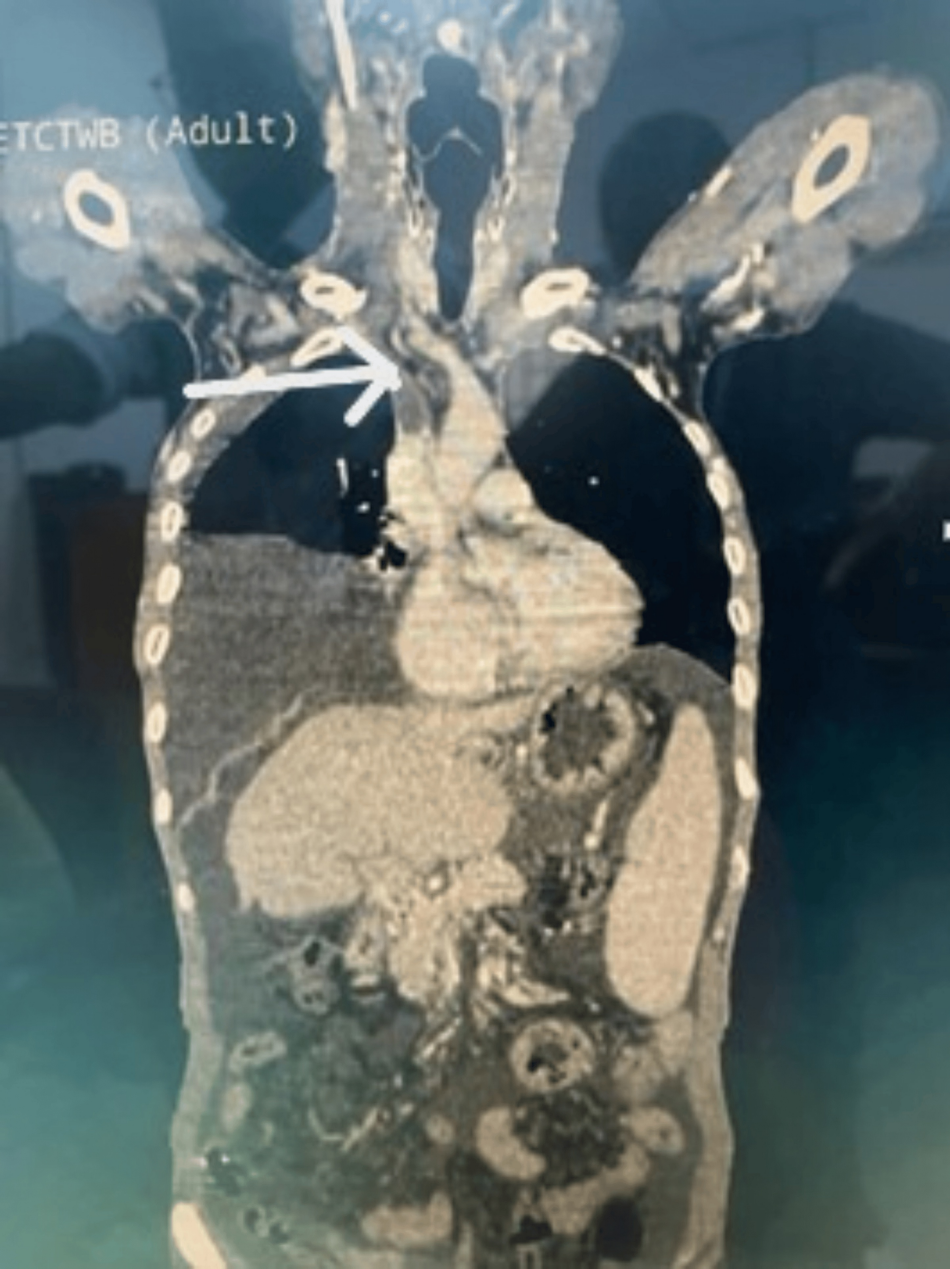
PET-CT showing right pleural effusion with subacute thrombosis of the right brachiocephalic vein, jugular vein, and proximal SVC (arrow) SVC, Superior vena cava; PET-CT, Positron emission tomography-computed tomography
